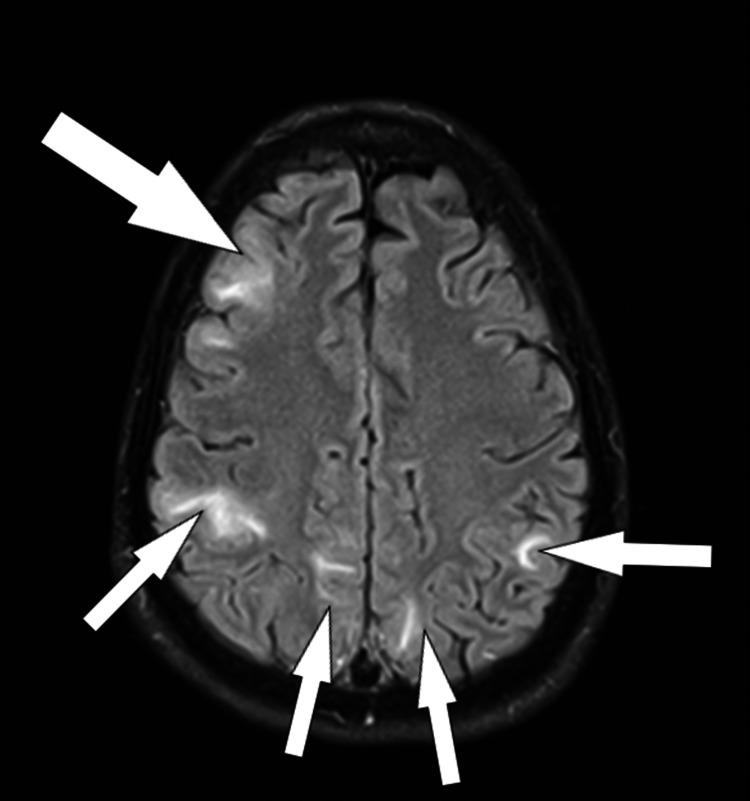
Another section of the same axial FLAIR MRI The arrows signal parasagittal gyriform hyperintensity with bilateral distribution FLAIR: fluid-attenuated inversion recovery; MRI: magnetic resonance imaging
